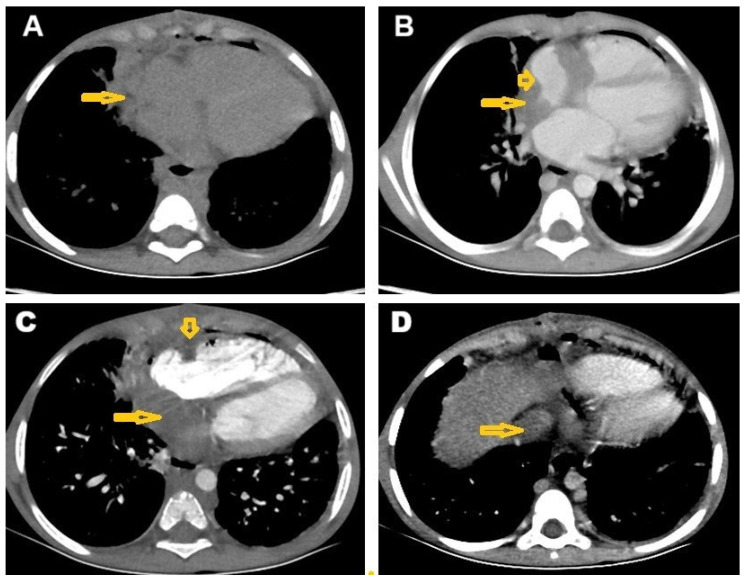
Selected cardiac computed tomography images. (A) Plain axial CT mediastinal window showing ill-defined right mediastinal infiltrative lesion around the right heart wall, and (B) post-contrast axial CT showing right mediastinal enhanced infiltrative lesion (arrows) around the right heart border that infiltrate the right atrium (short arrow). (C) Post-contrast axial CT mediastinal window showing ill-defined right mediastinal infiltrative lesion (arrow) around the right heart wall involving the right coronary artery (short arrow), and (D) post-contrast axial CT showing filling defect in the inferior vena cava (IVC) as a consequence of infiltration (arrows).
